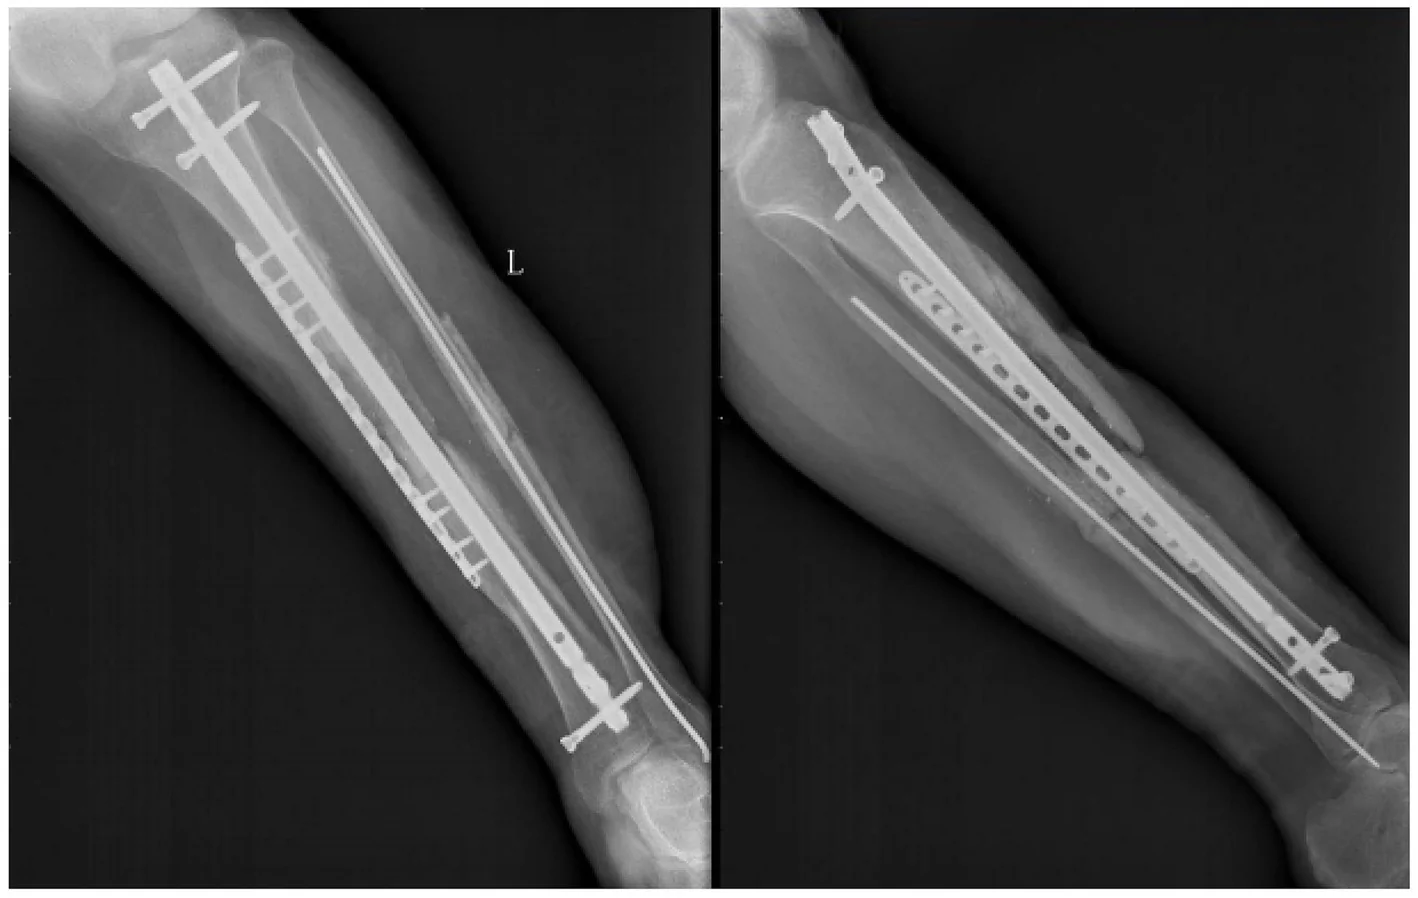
The X-ray findings three months after the bone grafting procedure, demonstrating partial bone sclerosis in the grafted area and cessation of bone resorption.
